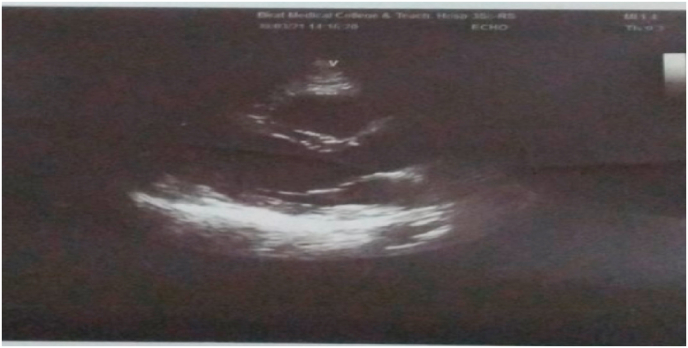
2D echocardiographic findings demonstrating minimal pleural effusion at presentation.
